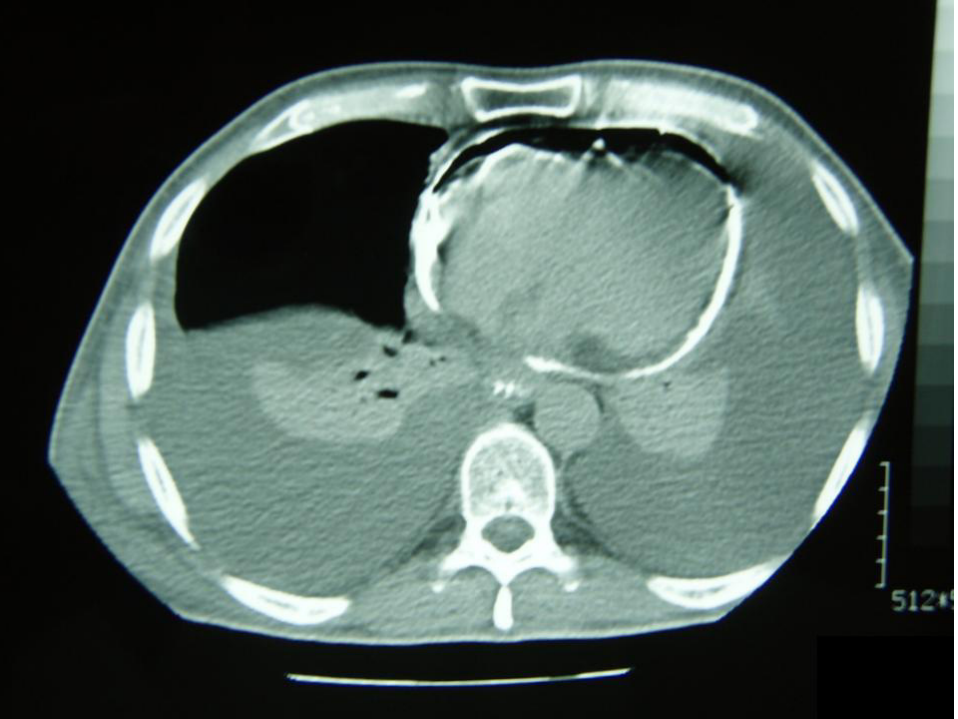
Thoracic computed tomography scan demonstrating hydropneumopericardium (air and contrast material filling the pericardial sac) and bilateral pleural effusions.
